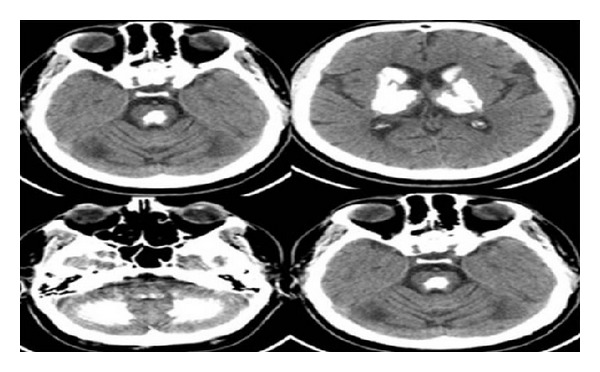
Brain computerized tomography (CT) scan: amorphous calcifications were detected in the bilaterally centrum semiovale, basal ganglia, capsula interna, thalami, mesencephalon, pons and bulbus, and the bilateral cerebellar hemispheres.
